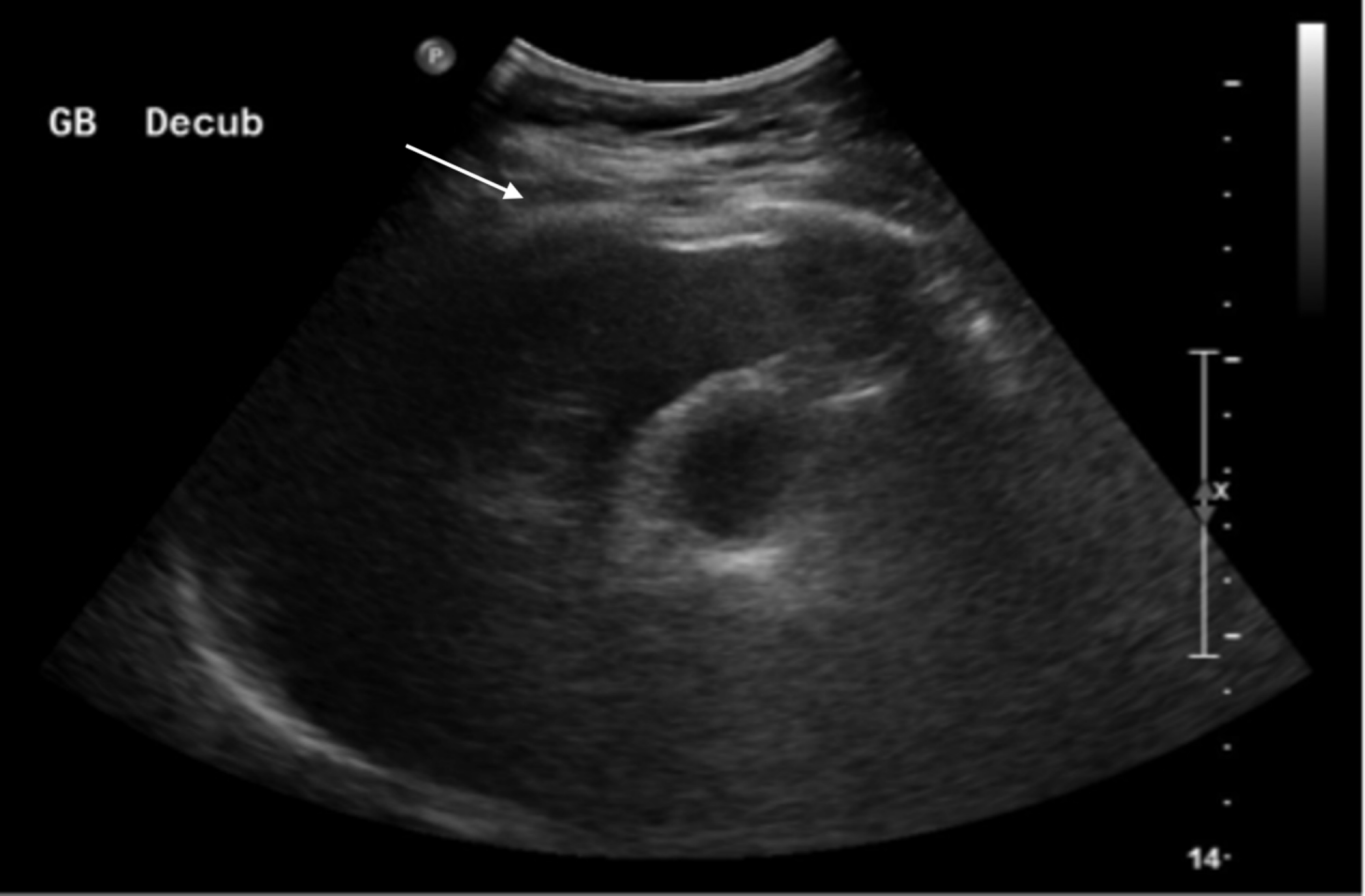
Abdominal ultrasound Gallbladder wall thickening (white arrow) with no evidence of gallstones or common bile duct dilation.
